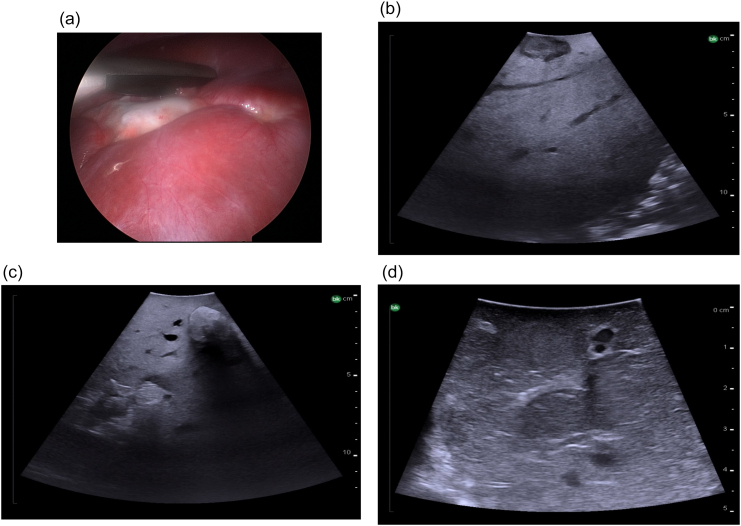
Intraoperative ultrasound appearance of colorectal liver metastases, a) Laparoscopic view of colorectal liver metastasis which is fibrotic, b) Hypoechoic metastasis with surrounding hypoechoic rim of fibrosis, c) Hyperechoic metastases, d) Isoechoic metastases.
